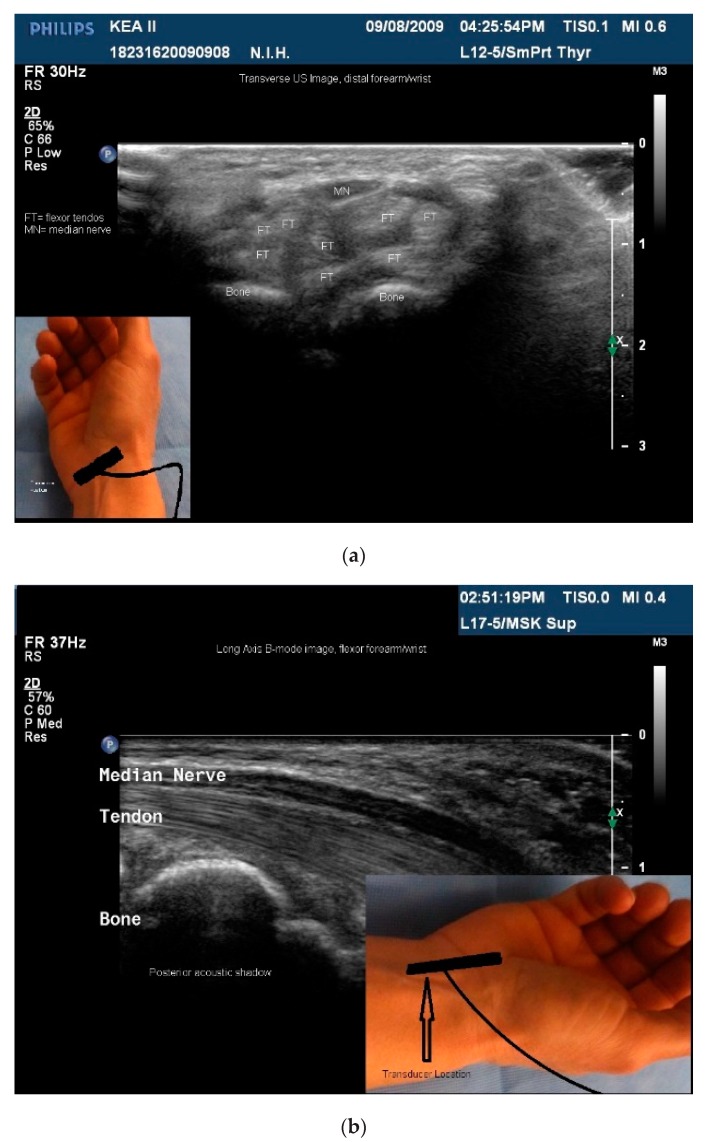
(a) Transverse B-mode ultrasound (US) image, distal flexor foream/wrist; (b) longaxis B-mode US image, distal flexor foream/wrist.
